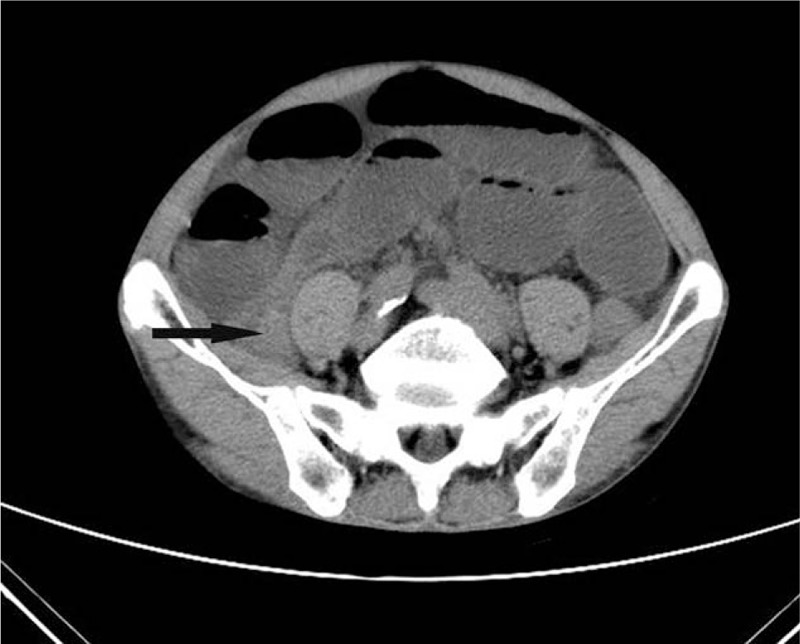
A CT scout of the abdomen revealed dilated small bowel loops with air-fluid levels suggestive of a small bowel obstruction. CT = computed tomography.
